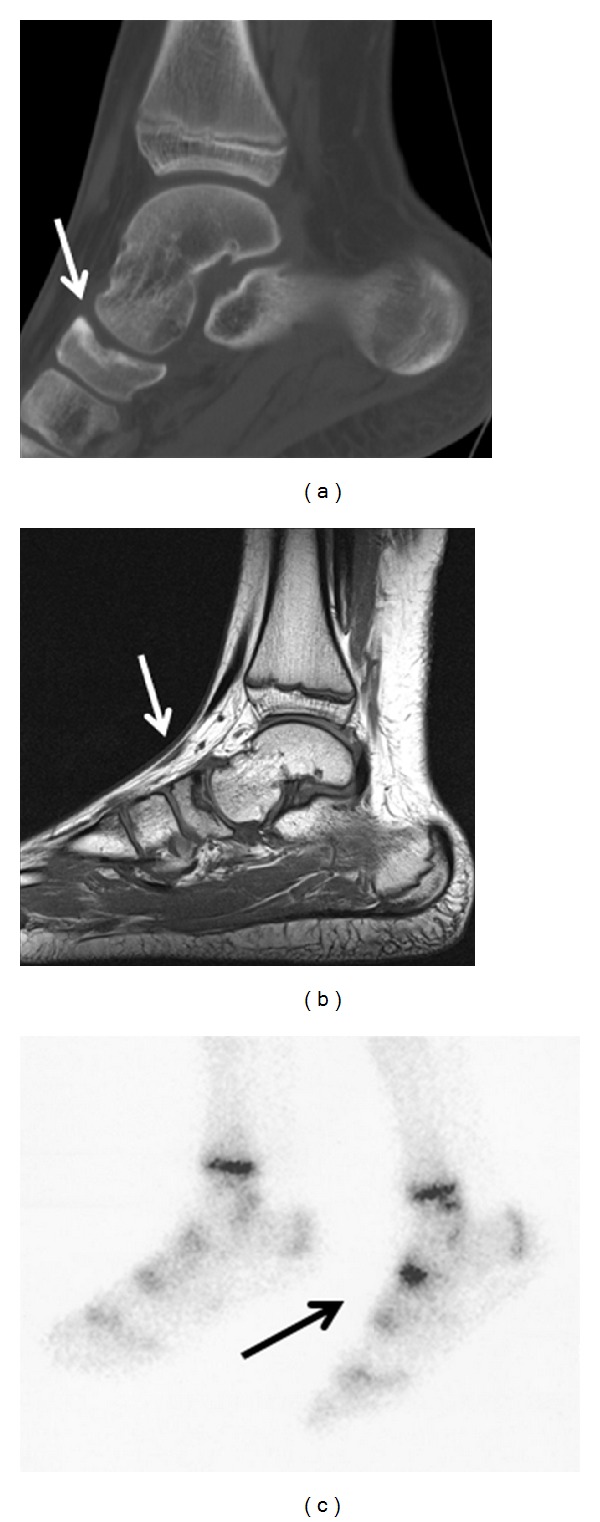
Left foot (a) CT scan: navicular bone indentation and subcortical lesion (arrow). (b) MRI: talonavicular joint effusion and irregularity of the proximal articular surface of the navicular bone (arrow). (c) Osseous scintigraphy: hyperfixation focus at the left navicular bone (arrow).
